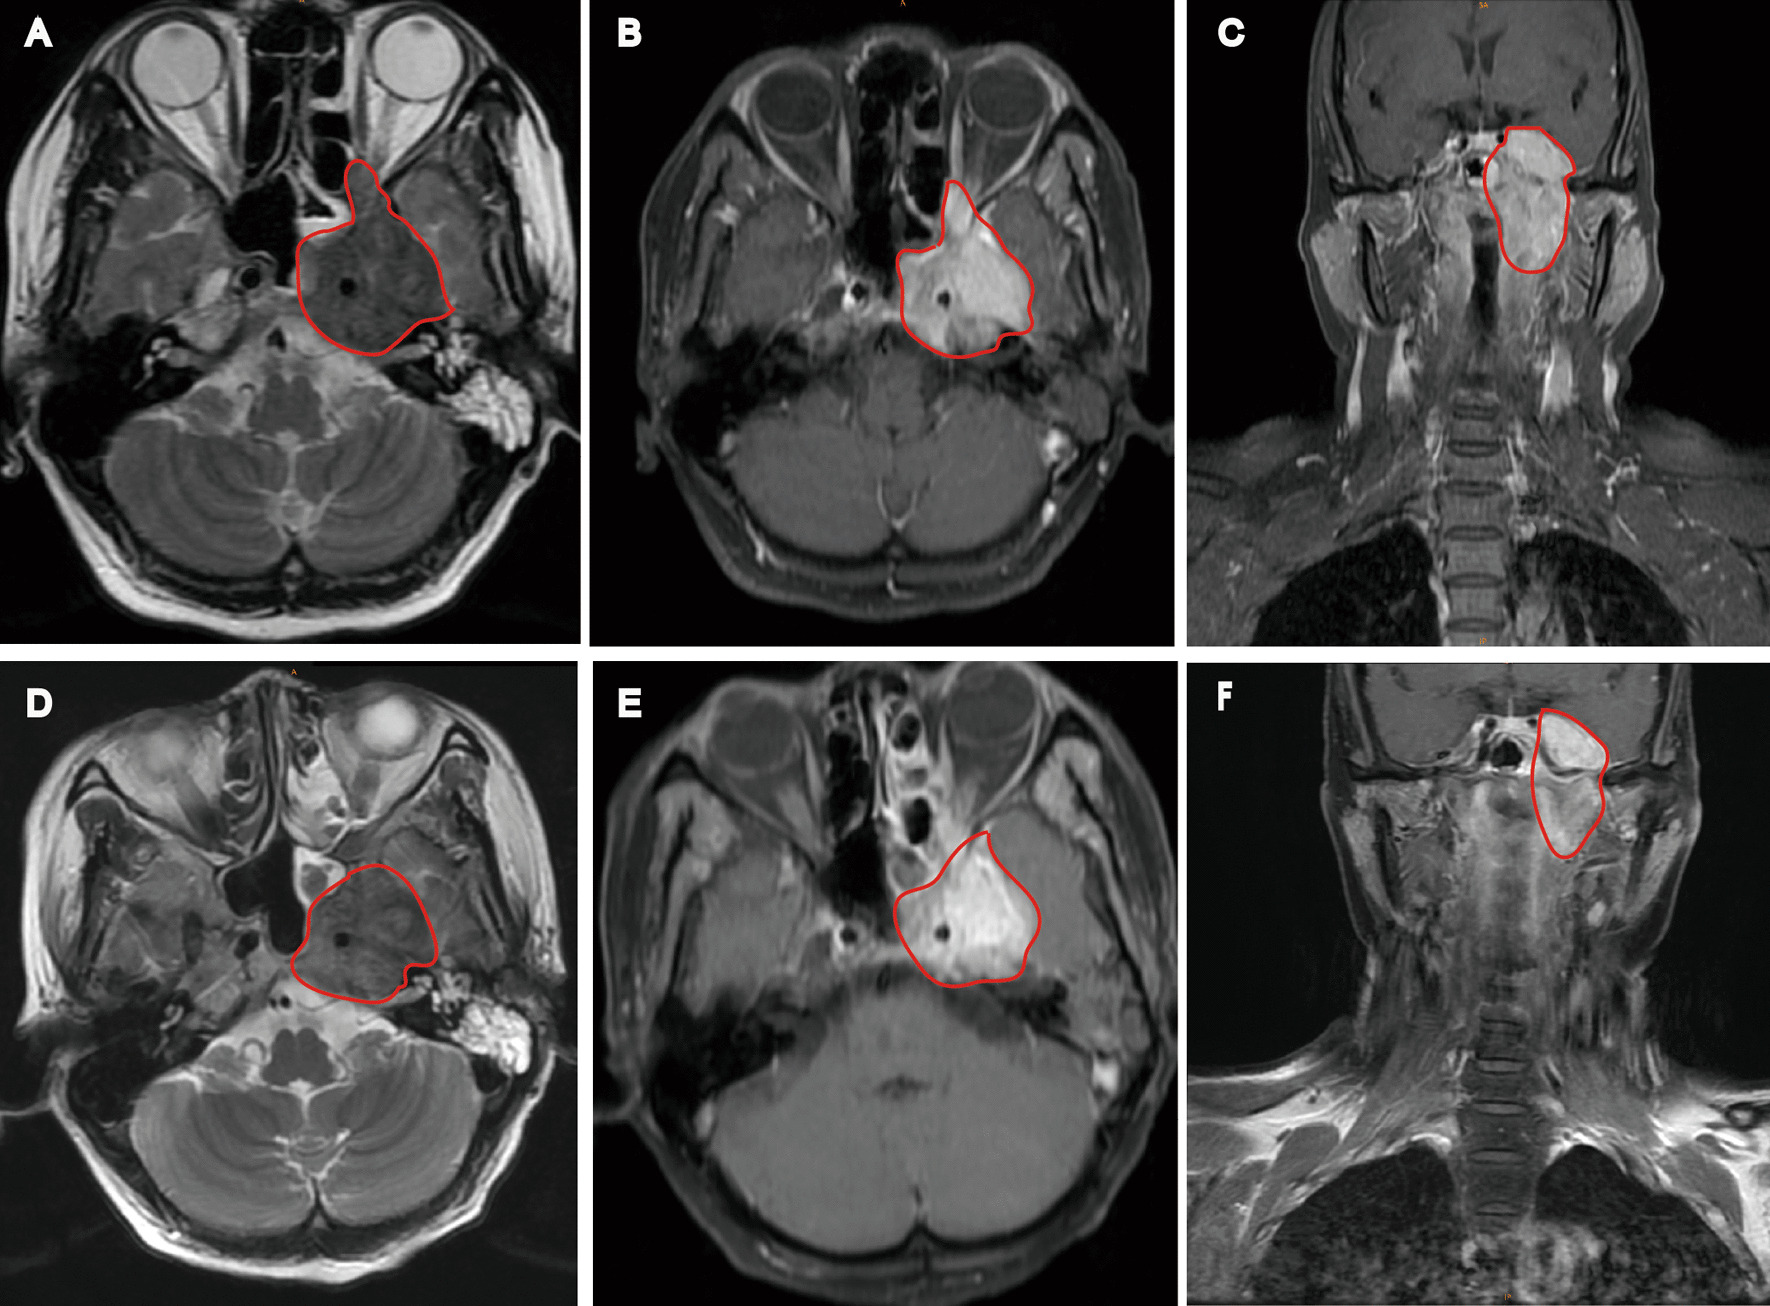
Pre-and post-treatment MRI of a 42-year-old female nasopharyngeal carcinoma patient with local residual disease after intensity-modulated radiation therapy. Pre-treatment MRI is shown in A–C; post-treatment MRI is shown in D–F. Post-treatment MRI indicated a local residual tumor in the nasopharynx, skull base, and intracranial space
